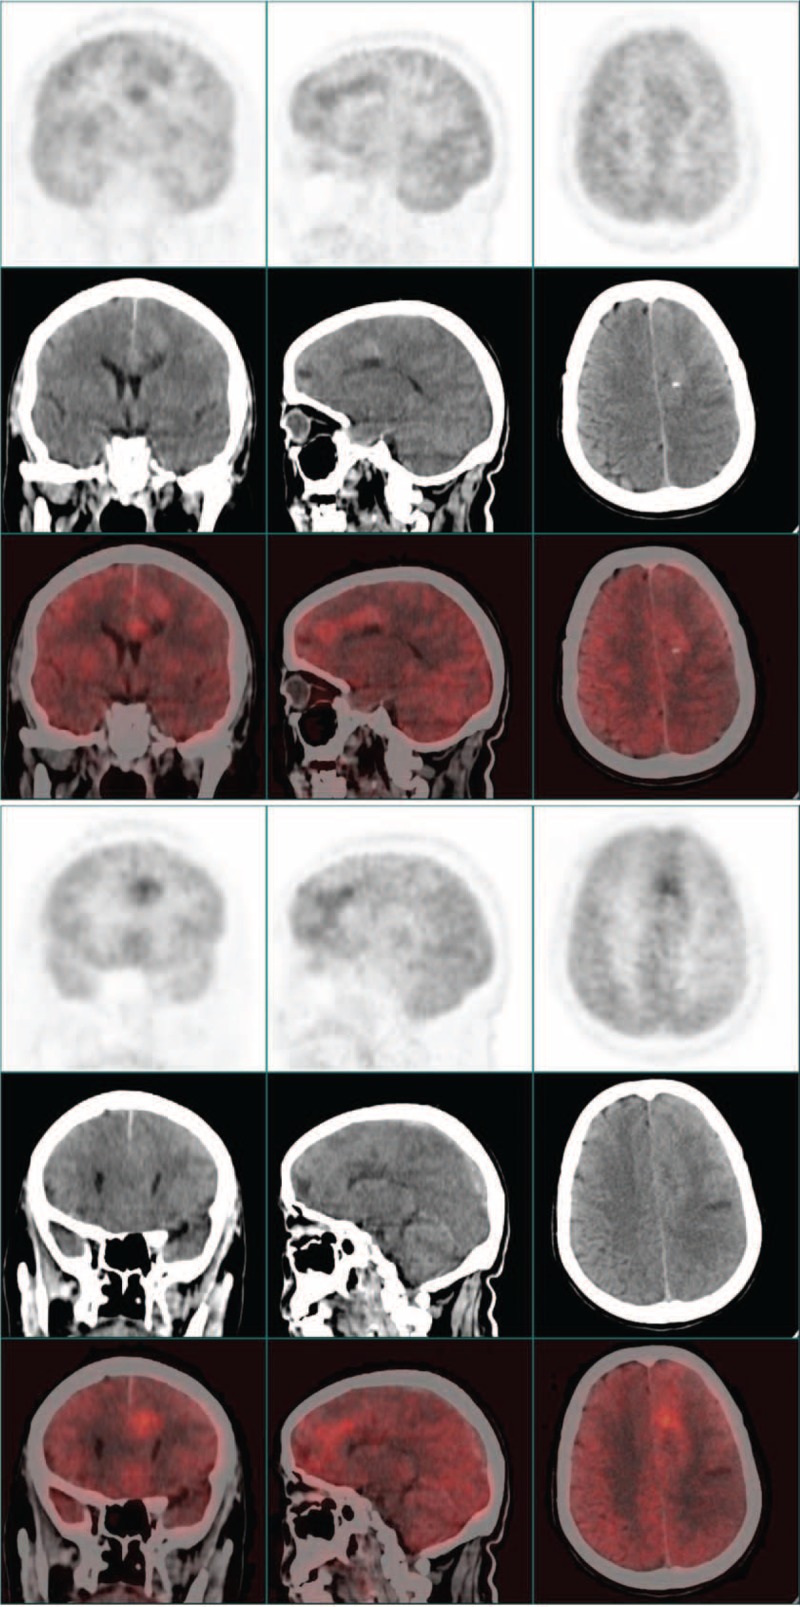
The figures of brain PET for this patient in different section levels. Multifocal irregular lesions with inhomogeneous high 18F-fluorodeoxyglucose (FDG) uptake were detected in left frontal lobe near centerline.
